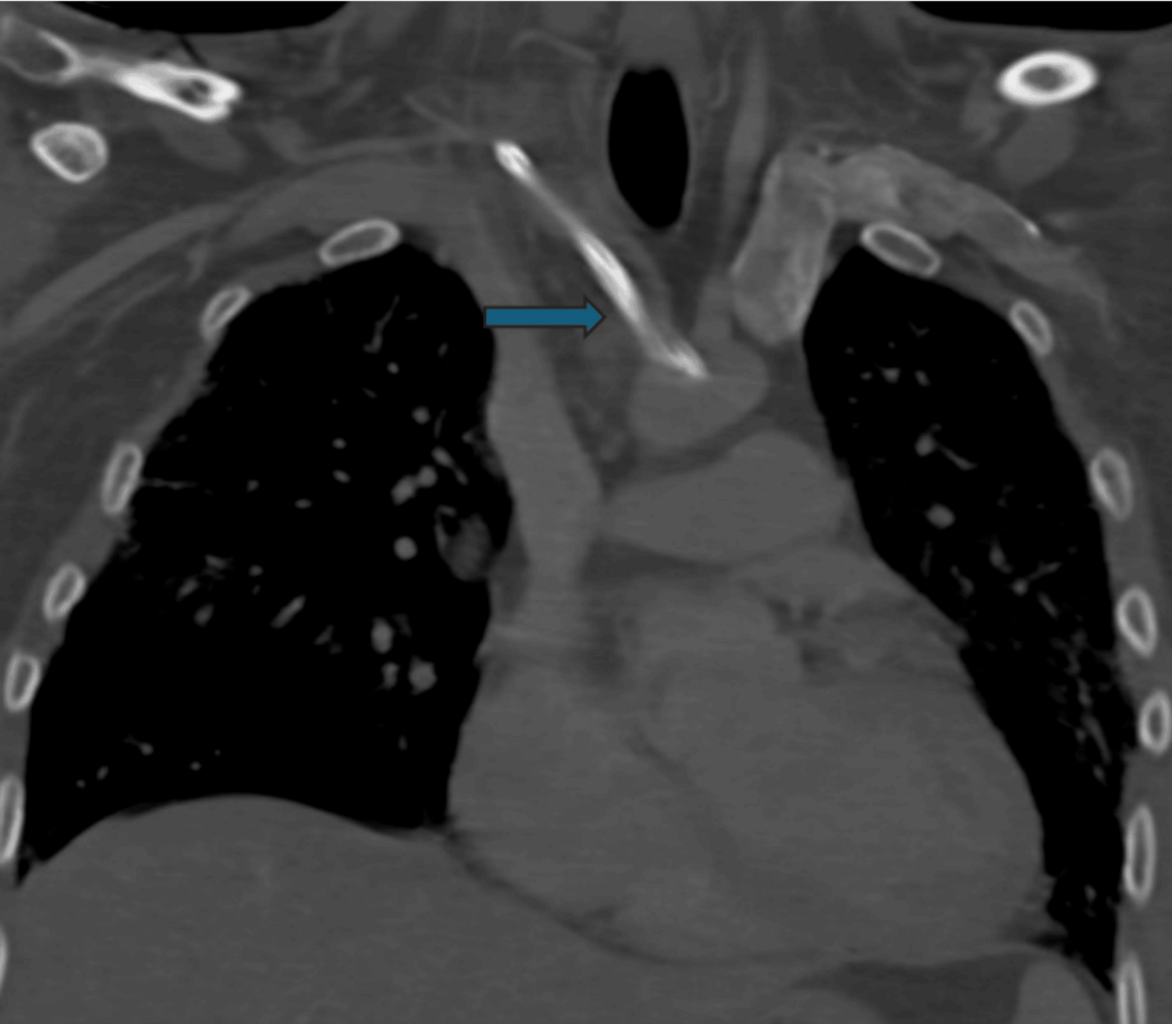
Computed tomography angiography (CTA) head/neck confirmed arterial placement of the central line (arrow).
